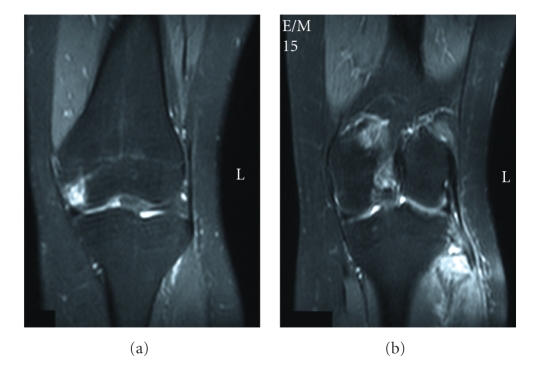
MRI of the left knee showing medial condyle bruise (a) as well as edema of the head of fibula due to fracture (b).
